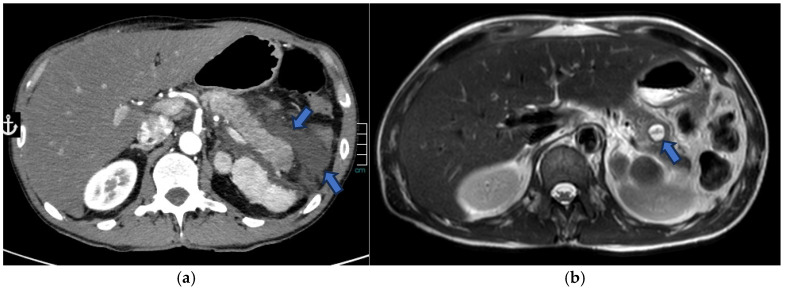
(a) Pseudocyst in a 49-year-old man: axial contrast enhanced CT scans show a free fluid collections surrounding the body and tail of the pancreas (arrow), which appears diffusely enlarged with loss of the normal lobulated contour. (b) MRI was performed four weeks later, demonstrating a well-defined, round cystic lesion located in the tail of the pancreas (arrow), which shows homogeneous high signal intensity on T2w images.
